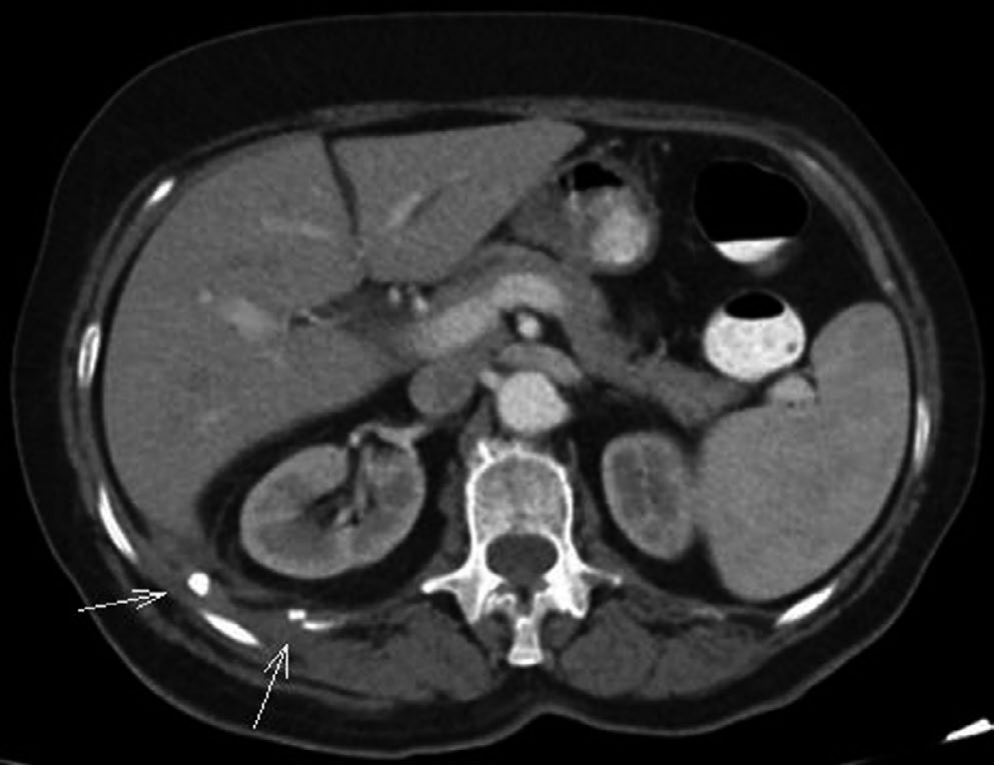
CT revealed the presence of two small 10 and 7 mm hyperattenuating lesions (white arrows) at the edge of the Morrison's pouch behind the upper pole of the right kidney with no evidence of intraabdominal and retroperitoneal abscess formation
